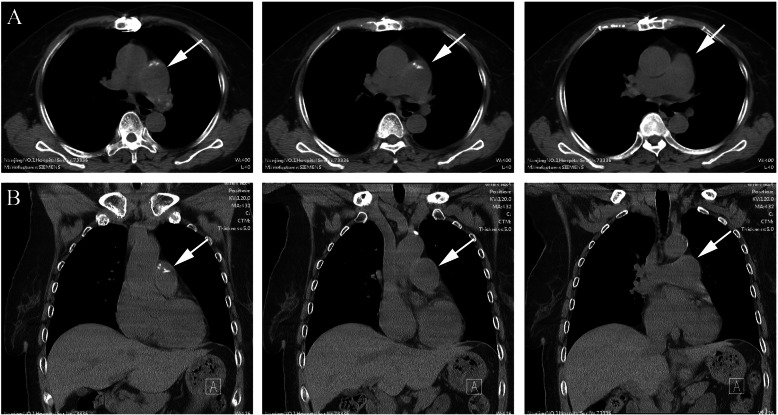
The chest CT showed partial high density shadow of pulmonary trunk and left branch, but no significant mass in the lung. a Transaxial, b Coronal, the lesion indicated by arrow
